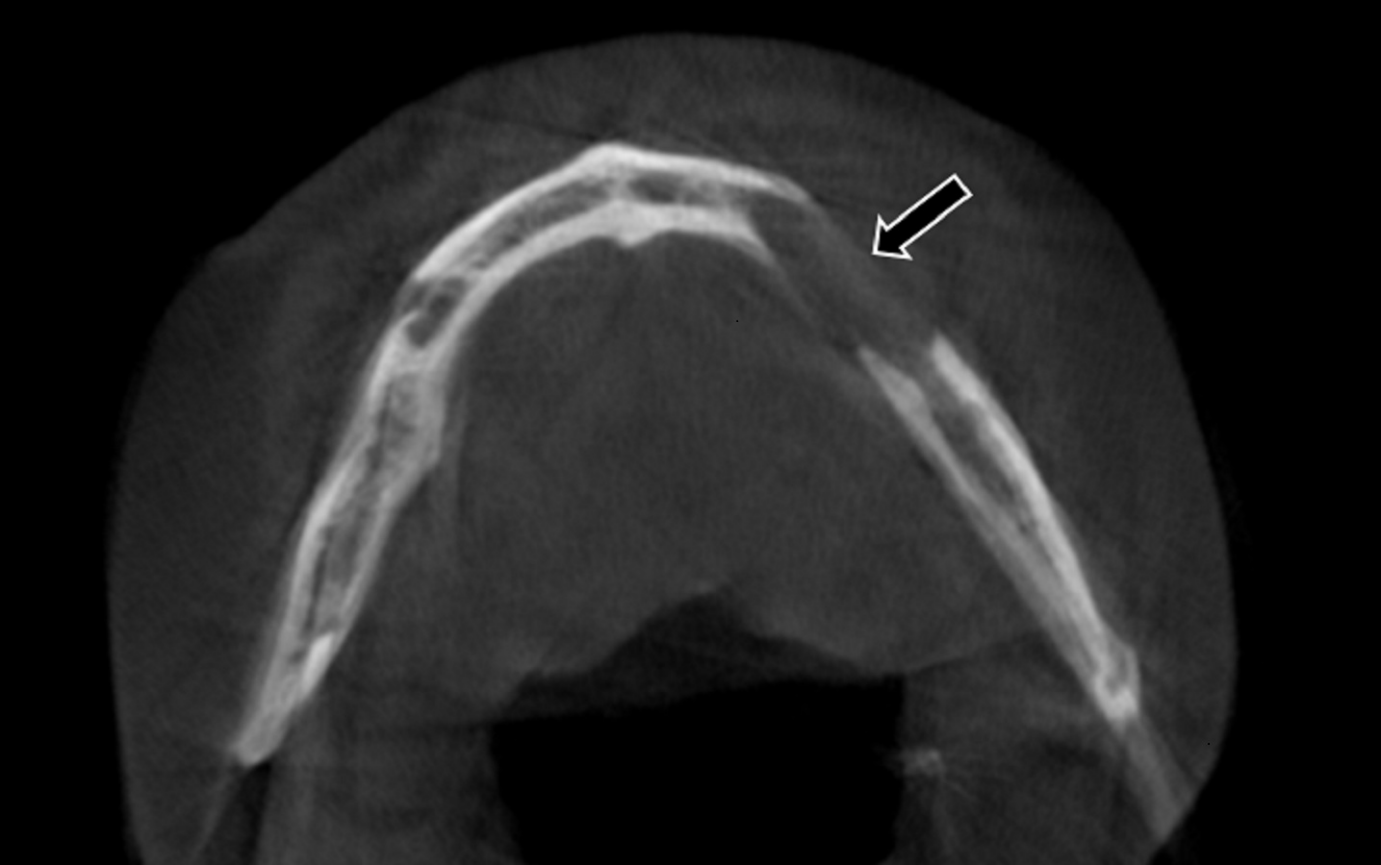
A cone beam computed tomography (CBCT) scan (axial view) showing an ill-defined radiolucent lesion in the premolar-molar region with perforation of buccal and lingual cortical plates (black arrow). CBCT - Cone Beam Computed Tomography
